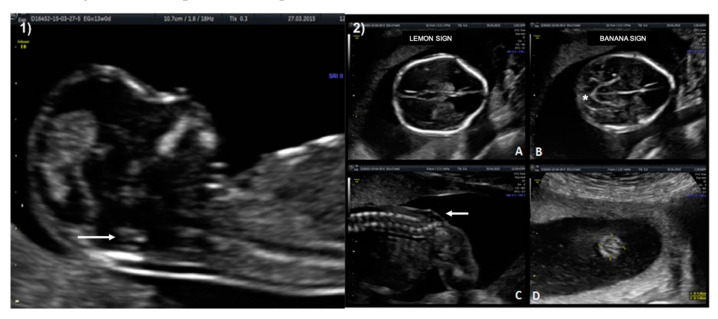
Case 2. First-trimester scan: (1) Ultrasound image in the mid-sagittal plane of the fetal face in a case of spina bifida aperta demonstrating compression of the fourth ventricle with no visible IT (white arrow). Second-trimester scan: (2.A) Lemon sign on axial plane at 19 weeks. (2.B) Banana sign in second-trimester scan (*), (2.C) Sagittal plane of the spine shows the neural tube defect between L5-S1 (white arrow). (2.D) Axial image from the sac.
